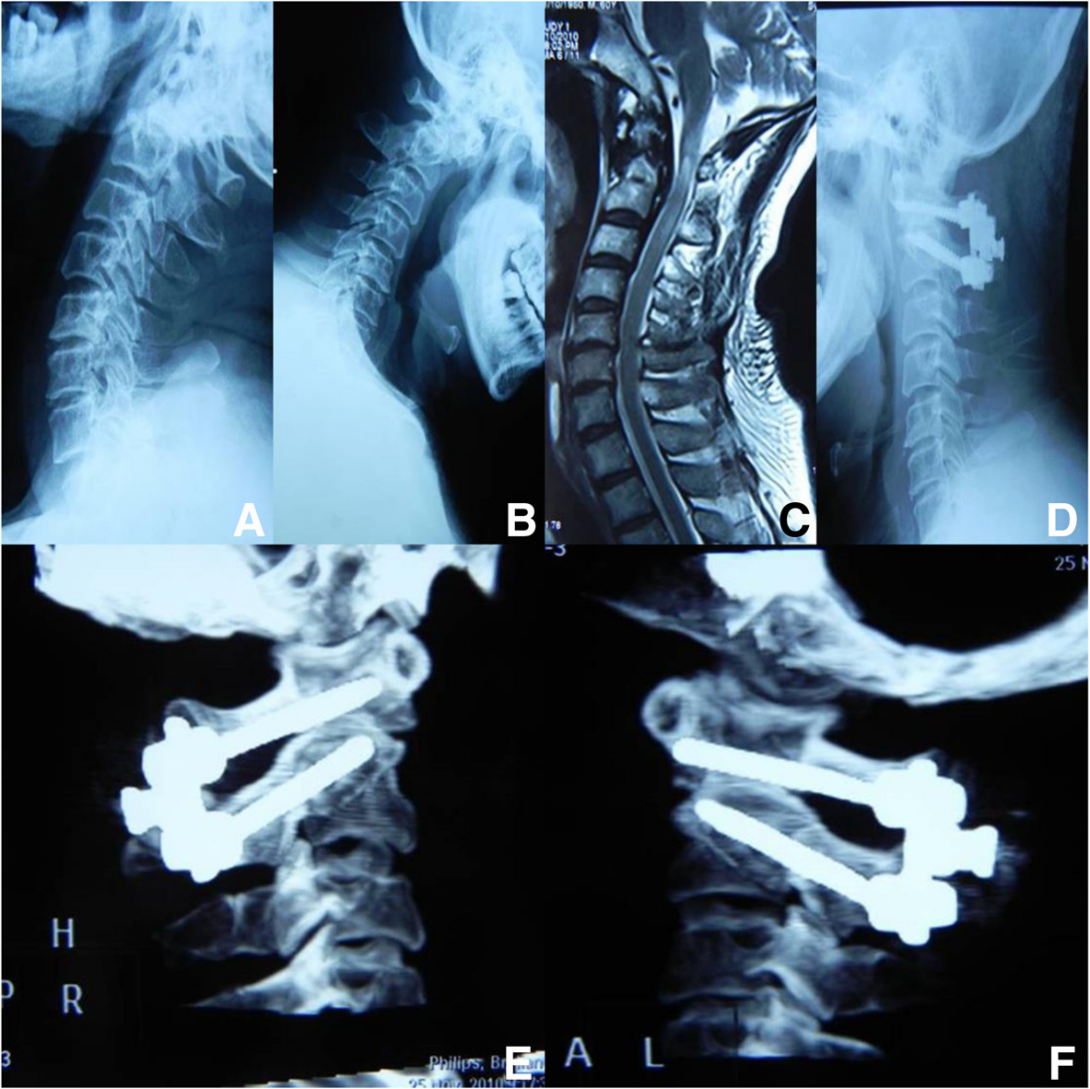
A patient who presented with atlantoaxial instability and cervical spinal cord injury underwent atlantoaxial fusion and internal fixation. a and b Dynamic radiographs showing atlantoaxial instability. c On MRI, the cervical spinal cord showing a hyperintense signal on T2-weighted images. d Lateral X-ray after surgery revealing good fracture reduction and internal fixation. e and f Postoperative sagittal reconstruction CT scans showing atlantoaxial internal fixation with atlas lateral mass screw and axis pedicle screw
